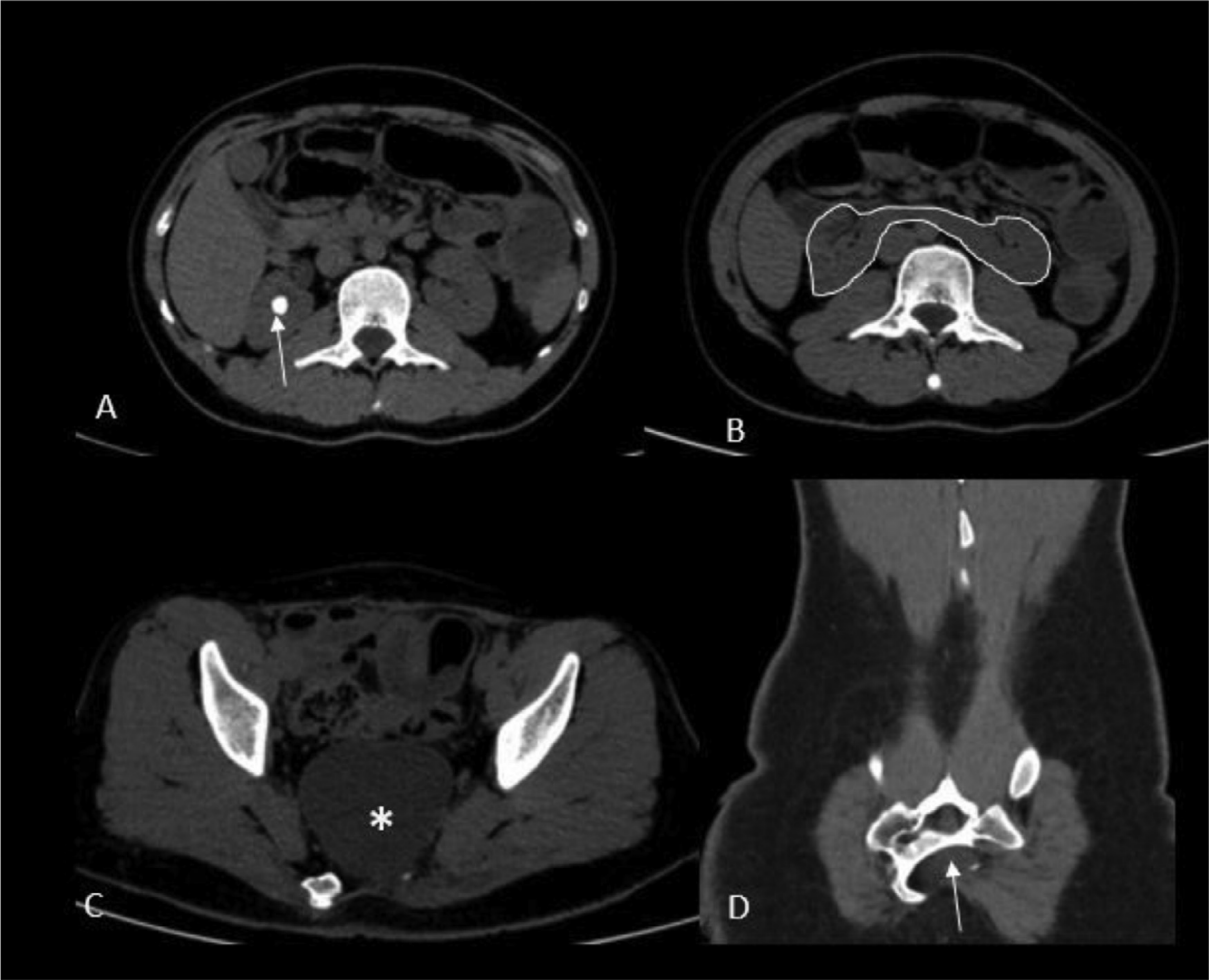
Axial non-enhanced CT scan shows right sided radiopaque renal stone (arrow, A). Horseshoe kidney (B) anterior sacral meningocele (*, C), and sacral dysgenesis (arrow, D).
